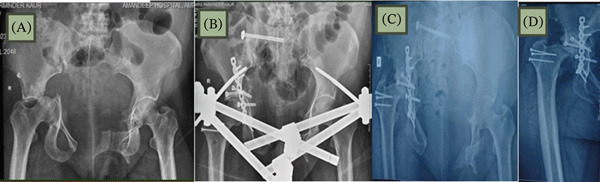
(A, B) Preoperative radiographs demonstrating right‐sided acetabular fracture with hip dislocation and sacroiliac injury treated with posterior wall plating, sacroiliac screw fixation, and a supra‐acetabular external fixator. (C, D) Normalized preoperative AP view with 20° internal rotation showing advanced hip osteoarthritis with femoral head deformity and subluxation, chronic pubic diastasis, and a Dorr type A femur.
